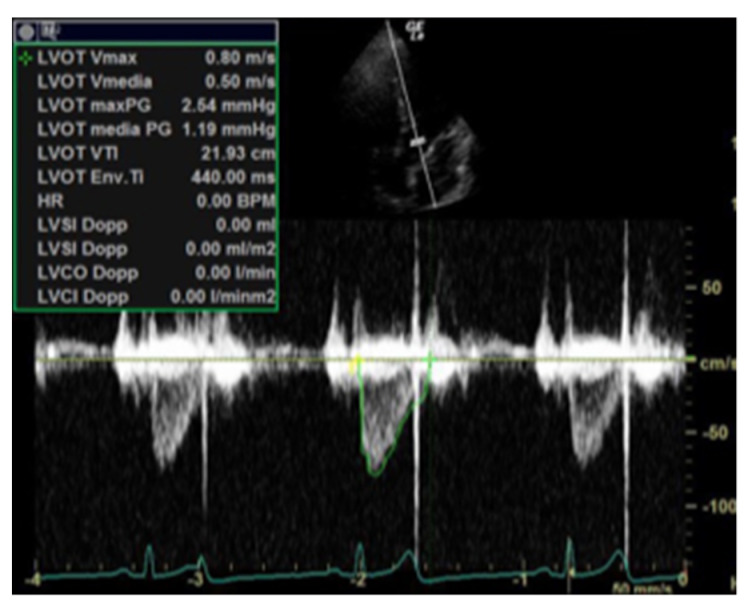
LVOT Velocity Time Integral (VTI), which is the distance the blood travels across the LVOT, calculated after tracing the LVOT pulsed−wave doppler.
